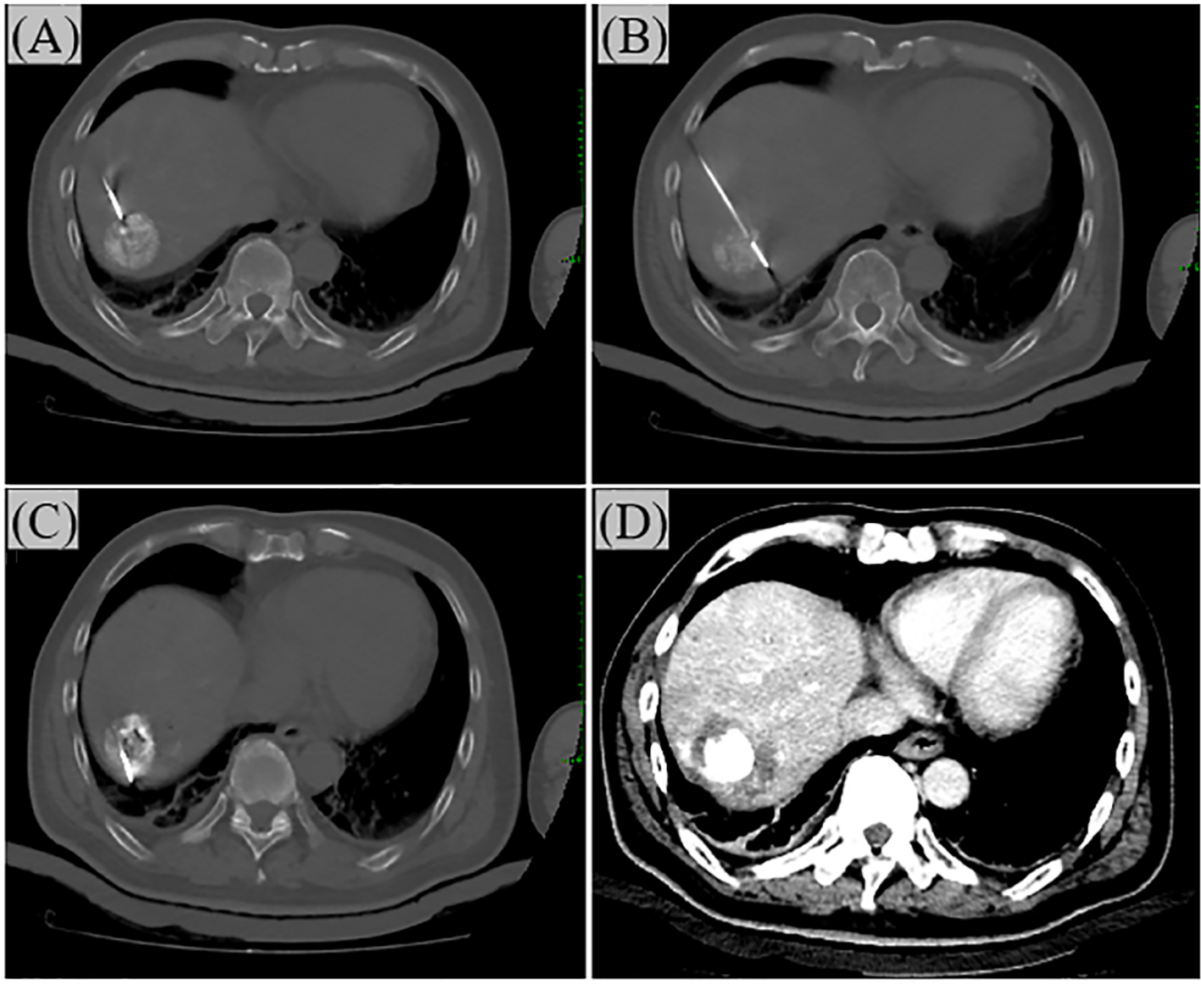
The operation process of Ablation. (A) Under CT guidance, the needle is inserted from the ventral side of the tumor. In the figure, the ablation needle has just entered the edge of the tumor. (B) Progressing step by step, the ablation needle enters the center of the tumor. (C) Insert the needle again to ablate the dorsal side of the tumor. (D) After the ablation is completed, perform a CT reexamination to ensure complete ablation. The ablation margin should be at least 0.5 cm.
